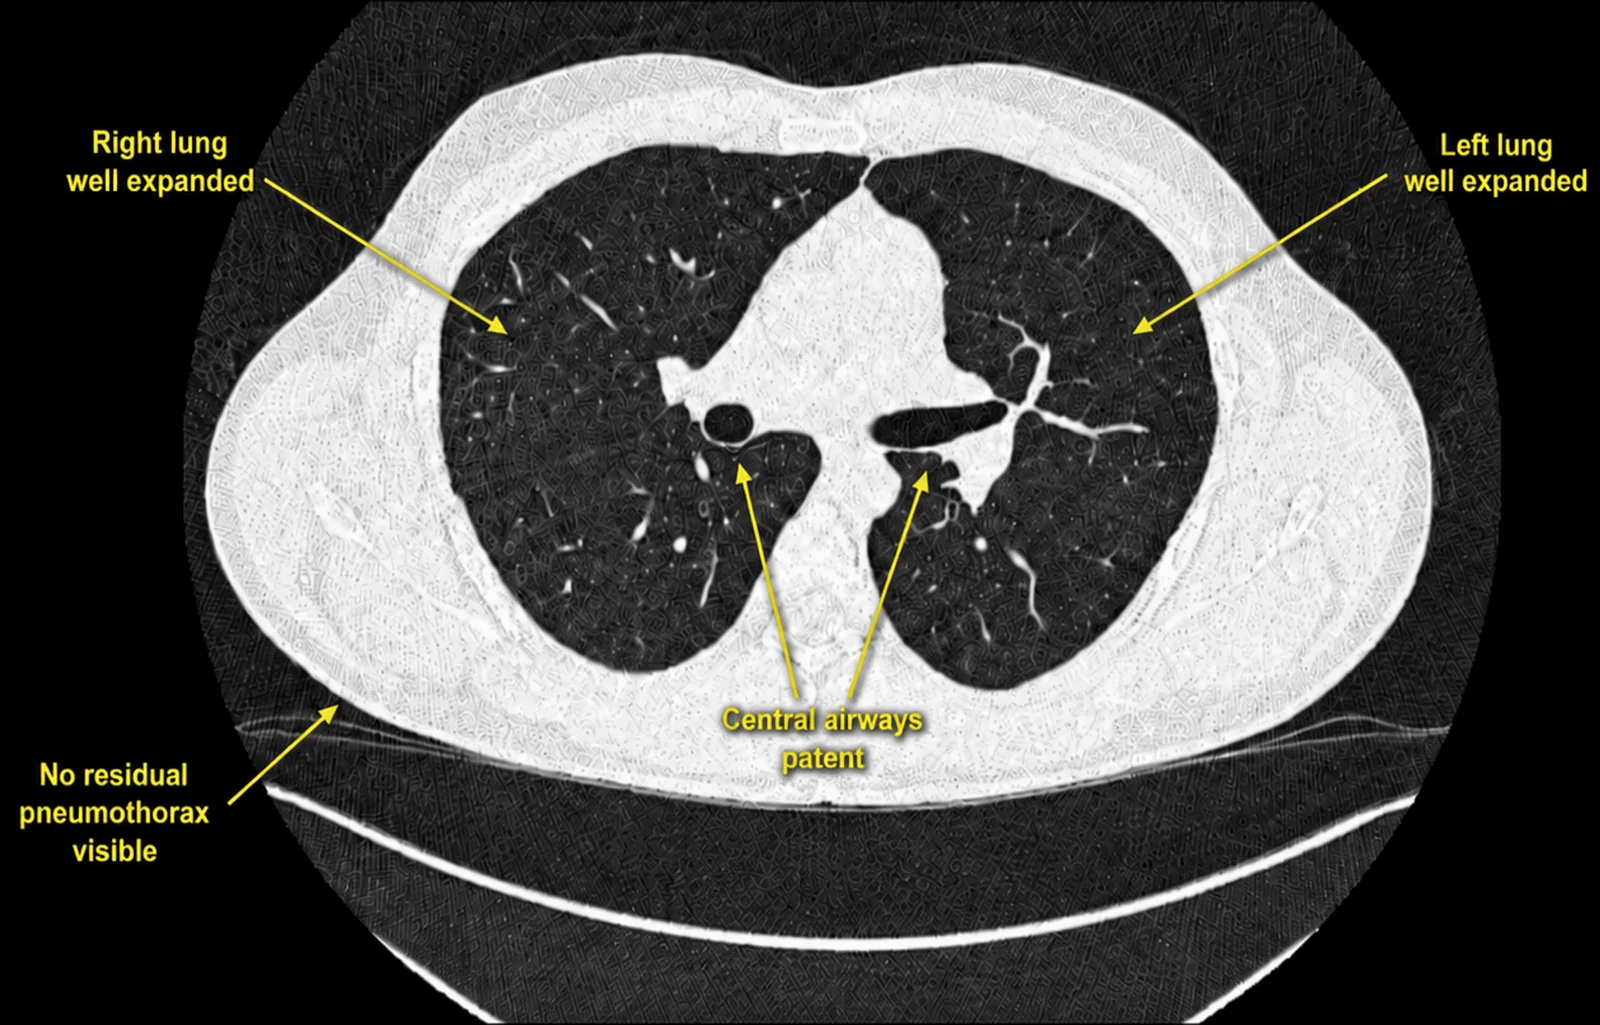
Follow-up CT of the thorax: complete resolution of the previously seen small bilateral pneumothoraces; both lungs are well expanded, with no evidence of underlying bullous or cystic lung disease, focal lung mass, lymphadenopathy, pleural effusion, or parenchymal destruction CT: computed tomography
